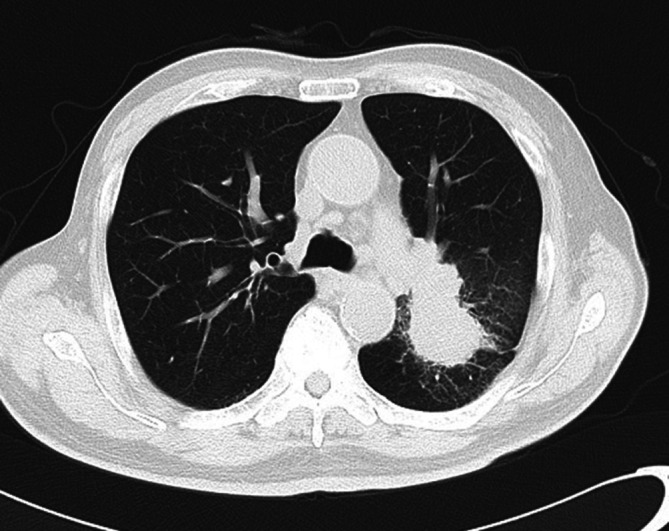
Chest computed tomography (CT) showing the tumour located in the left S1 + 2c segment with invasion into the lower lobe.
